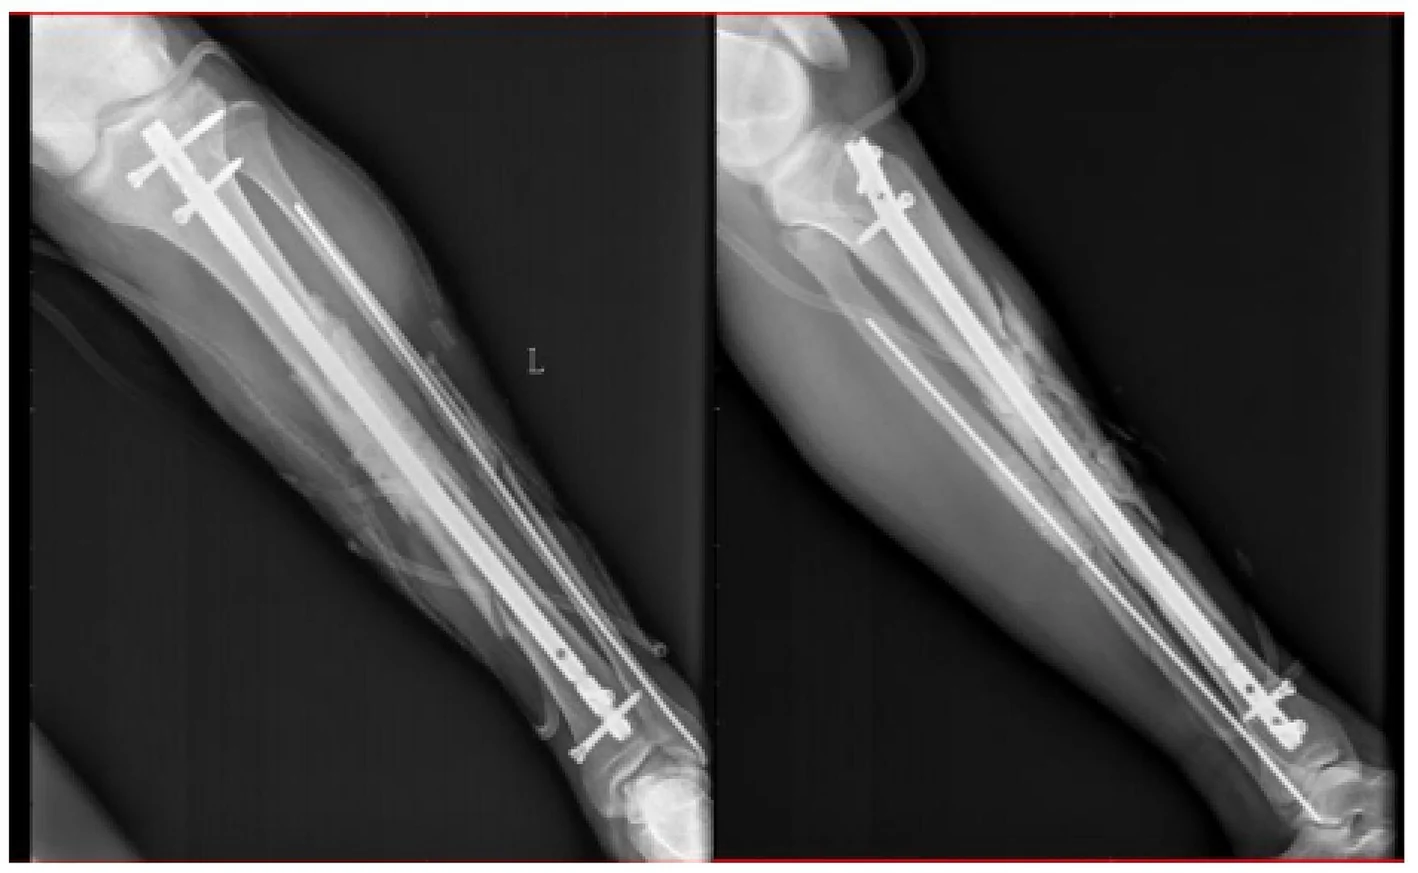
After the third debridement post-admission, the external fixator was replaced with internal fixation using antibiotic-coated intramedullary nailing technique. The bone defect area was again protected with antibiotic bone cement spacer. This image shows the immediate post-operative X-ray of the affected limb.
